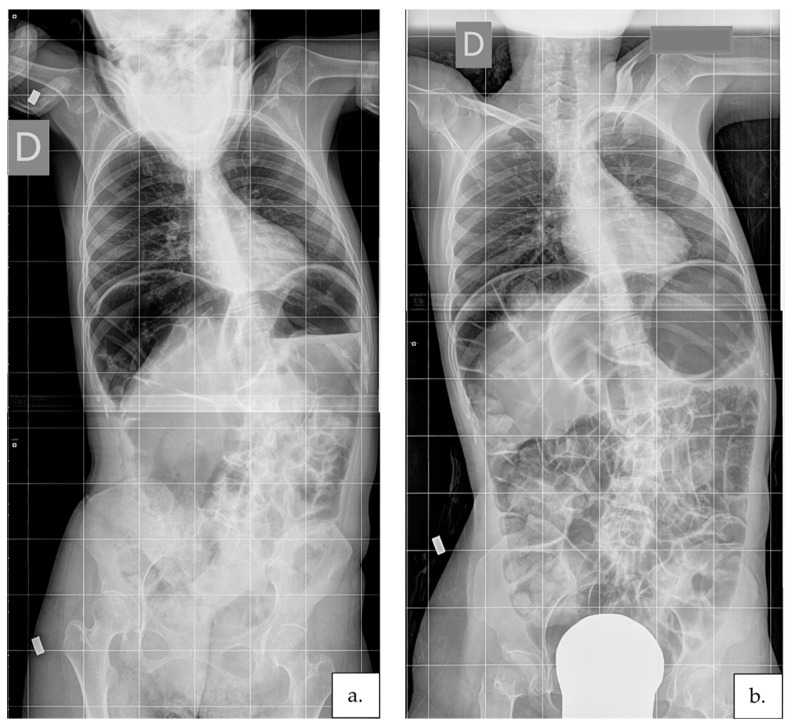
Participant no. 11′s radiographs collected before (a) and after (b) the intervention. She was 31.6 years old at T1 and used to sit with trunk supported for most of her waking hours. She was able to stand and walk only with significant support given at the trunk from behind. During the intervention, she started a daily program of unsupported asymmetrical sitting, walking on a treadmill, and overcorrecting passive posture maintenance. At the end of the intervention, she was able to walk more than 10 steps and stand for more than one minute with support given to the distal section of both hands. An improvement of 11° Cobb was recognized at T2.
